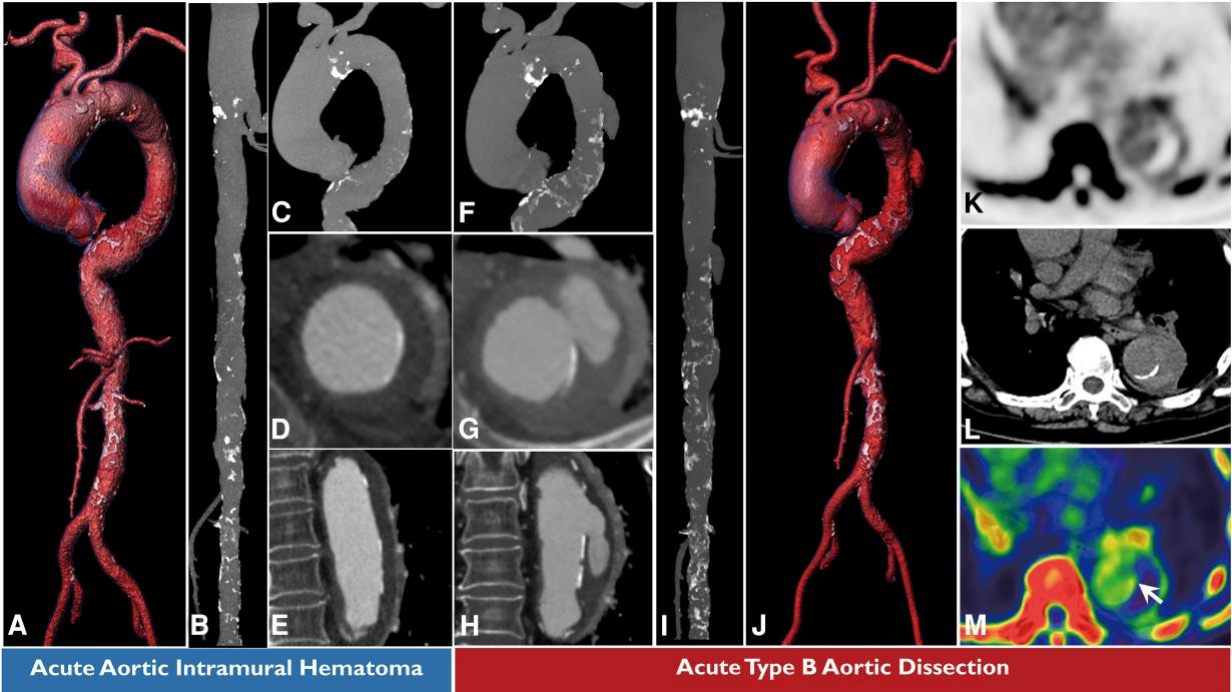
(*A*) Three-dimensional volume rendering of computed tomography angiography of the previously acute aortic intramural haematoma. (*B–C*) Three-dimensional volume rendering of the stretched aorta and thoracic aorta. (*D*) Reconstructed axial image (perpendicular to the aortic centre lumen line) prior to the intimal tear formation. (*E*) Sagittal image of the aortic intramural haematoma. (*F*) Three-dimensional volume rendering of computed tomography angiography of the thoracic aorta after dissection. (*G*) Reconstructed axial image (perpendicular to the aortic centre lumen line) to show the intimal tear. (*H*) Sagittal image of the post-dissected aorta. (*I–J*) Three-dimensional volume rendering of computed tomography angiography of acute aortic dissection. (*K*) ^18^F-sodium fluoride uptake at the site of intimal tear on positron emission tomography image. (*L*) Axial image at the site of intimal tear on computed tomography image. (*M*) Pseudocolour fusion of positron emission tomography and computed tomography images at the same axial plane of the intimal tear. PET, positron emission tomography; CT, computed tomography.
